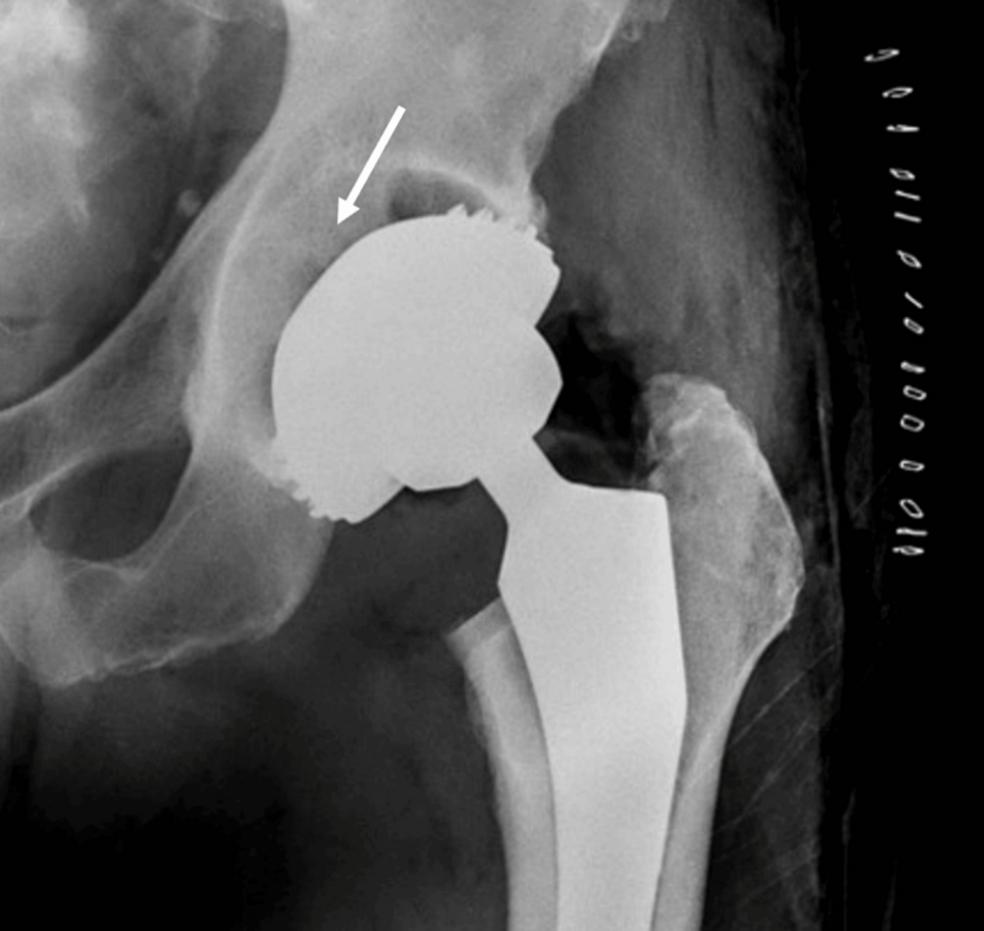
Anteroposterior X-ray view of an incomplete threaded cup placement The white arrow shows the space between the cup and the acetabulum roof. The threads have fixated at the osteophytes of the peripheral rim
